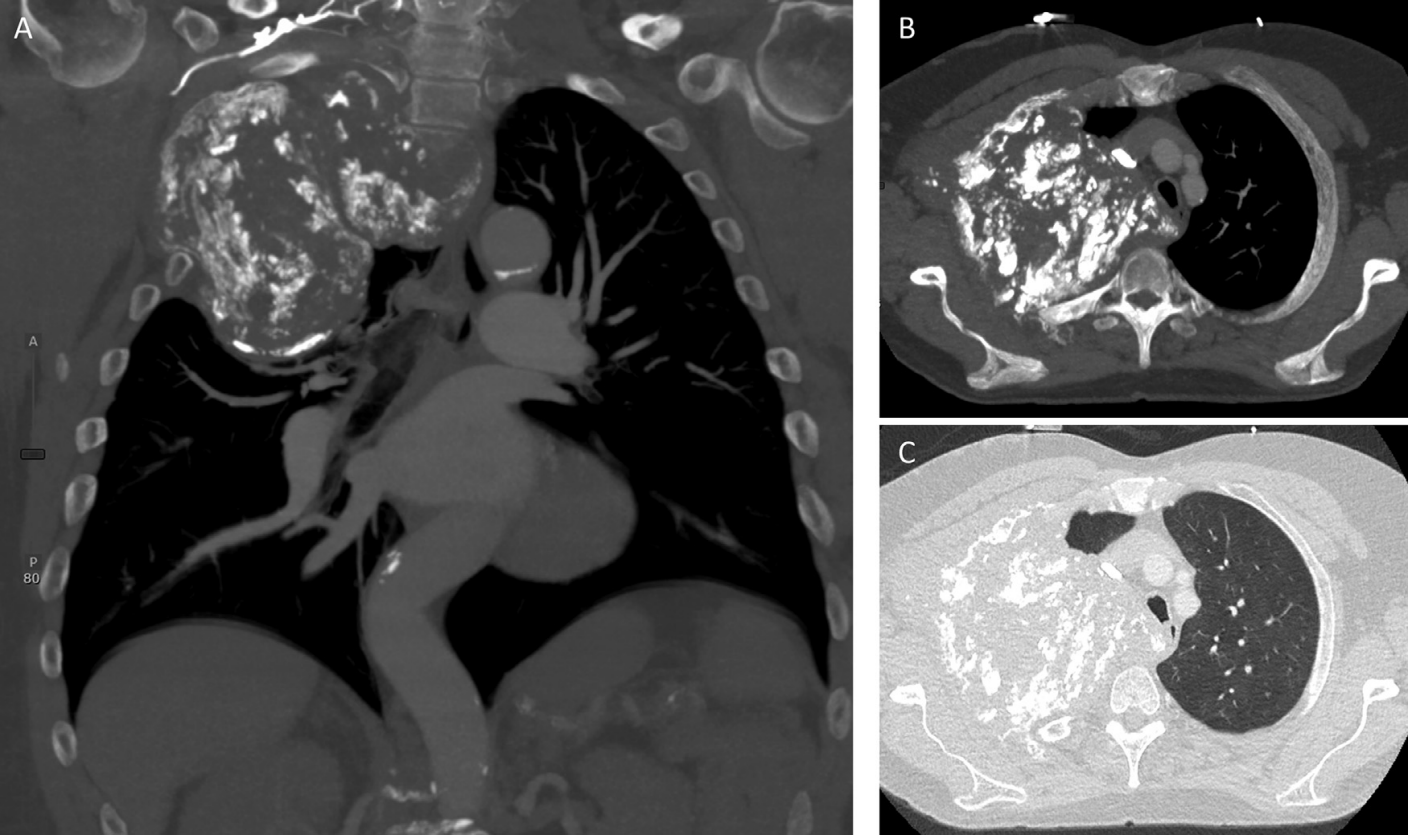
Contrast-enhanced CT of the chest, coronal (A), mediastinal window axial (B) and lung window axial (C) demonstrates no abnormal enhancement in right upper chest wall mass that extends through the pleura into the right axilla and it erodes the right second rib
